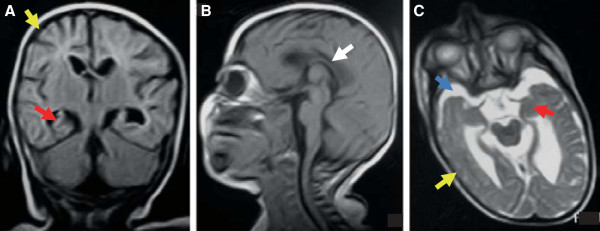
Brain MRIs of II:4. A Supratentorial atrophy with thin cerebral cortex (yellow arrow) and hippocampal dysplasia (red arrow) are visible in the coronal plane. B Hypoplasia of corpus callosum. Note craniofacial dysproportion and sloping forehead (sagittal MRI). C Hypotrophic temporal lobes with widened subarachnoidal space (blue arrow), hippocampal malformation (red arrow) and gyral pattern anomaly (axial MRI).
